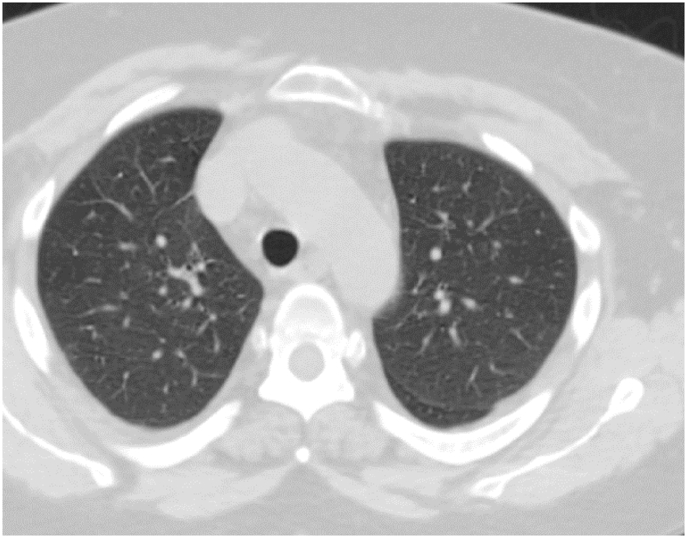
CT chest (lung window), December 2020: normal CT chest.
